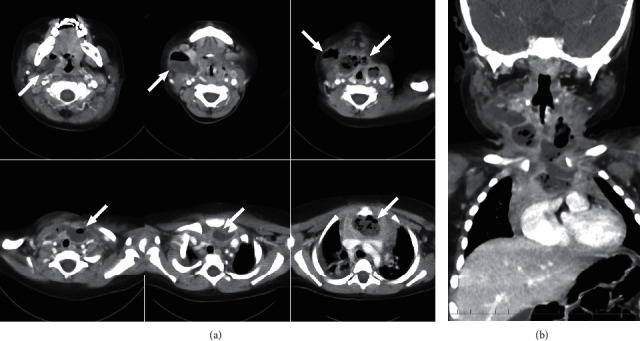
Axial (a) and coronal (b) images from the contrast-enhanced CT scan of the head and chest on admission. The white arrows show an abscess ranging from the neck to the anterior mediastinum.
